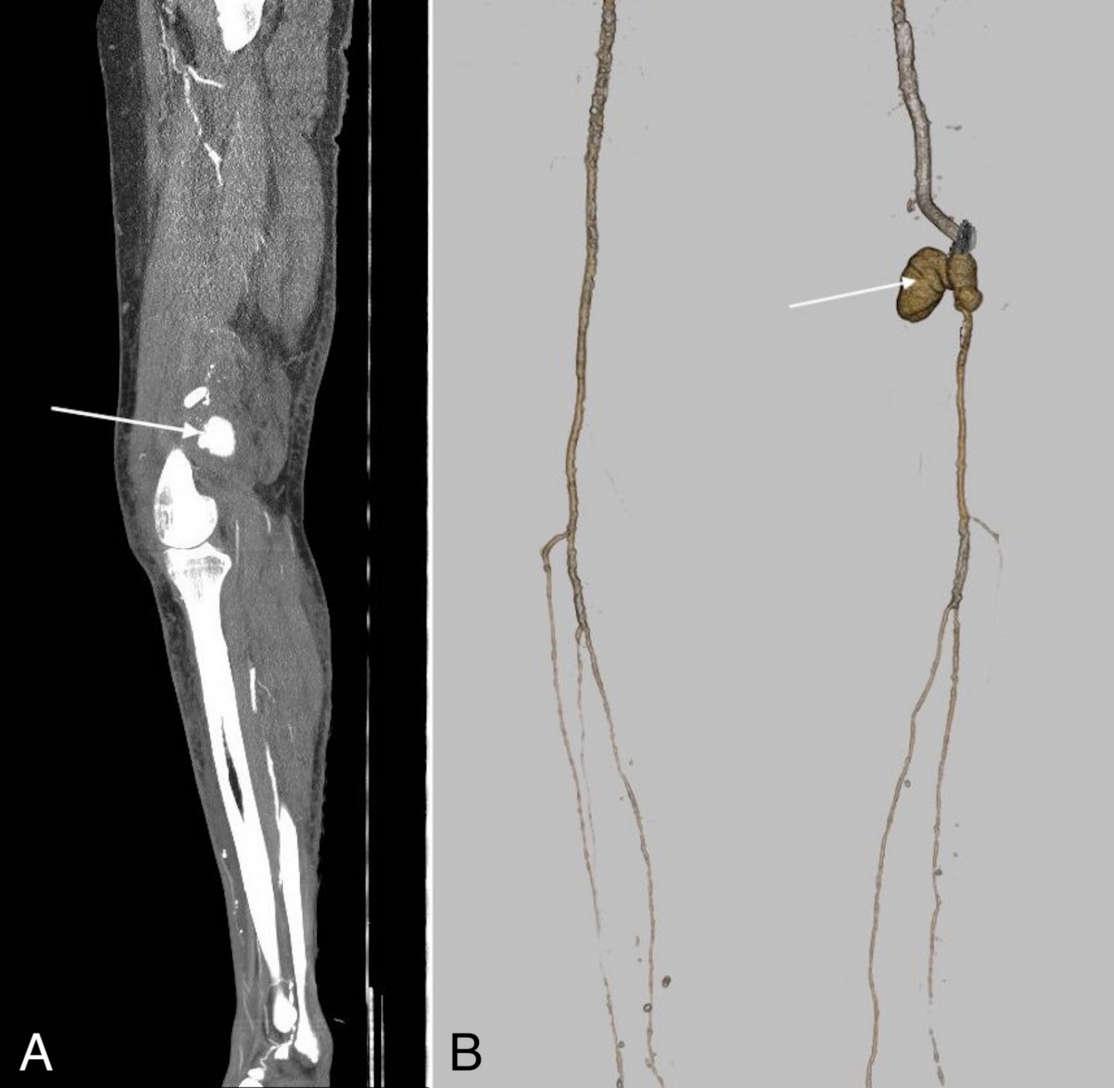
(A) CTA of the left leg shows a pseudoaneurysm (arrow) in the popliteal fossa. (B) A 3,5 x 3,5 cm pseudoaneurysm (arrow) behind the caudal end of the stent-graft placed in the SFA can be observed on the reconstruction. SFA - Superficial femoral artery, CTA - Computed tomography angiography
